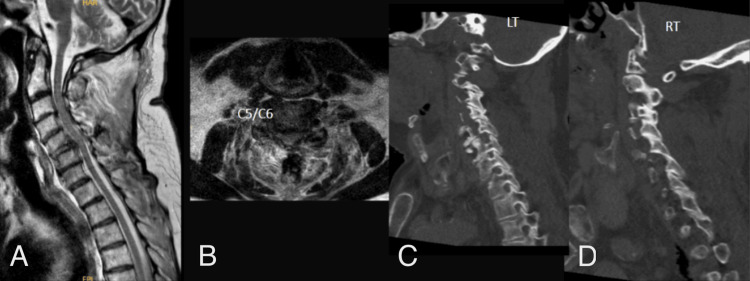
C5-C6 fracture with associated disc injury and prevertebral hematoma, selected for Type 1 MICEPS following ACDF. (A) Mid-sagittal T2 MRI; (B) axial T2 MRI view of the index level; (C) paramedian left view, CT scan; (D) paramedian right view, CT scan. MICEPS, minimally invasive cervical pedicle screw; ACDF, anterior cervical discectomy and fusion
